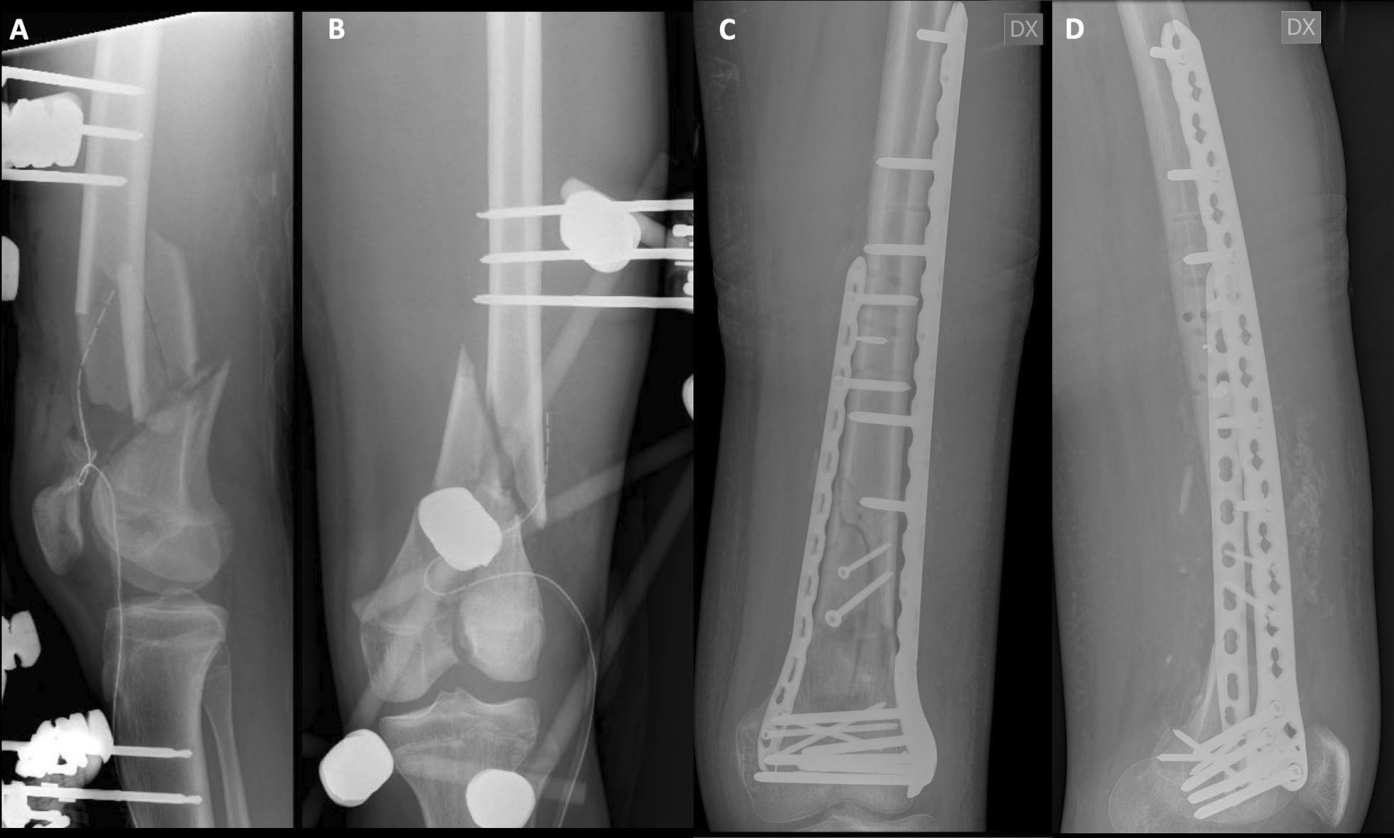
Preoperative radiographs in lateral view (A) and anteroposterior view (B), and postoperative radiographs in anteroposterior view (C) and lateral view (D) of a patient treated with double plating
